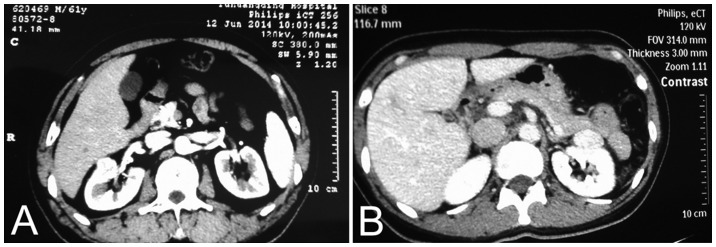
Renal computed tomography scans showing tumors located at the (A) right renal hilum and (B) left renal hilum.
